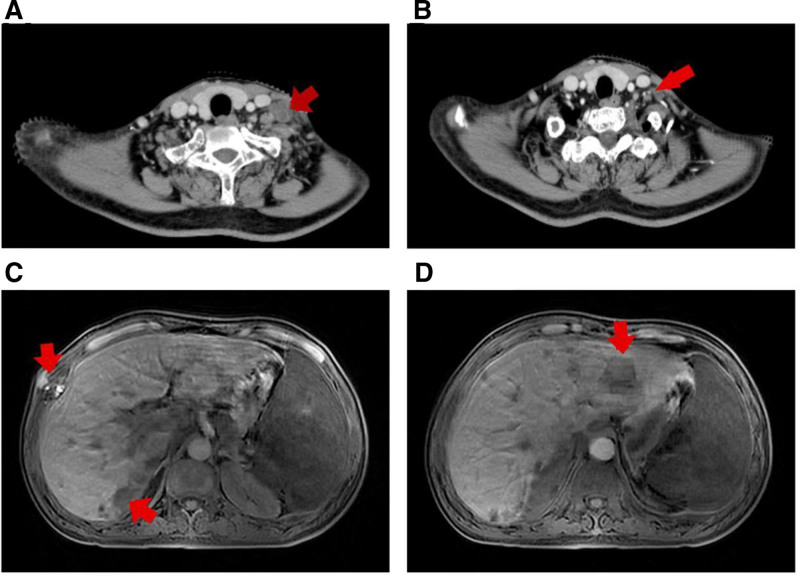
(A) Cervical lymph nodes before radiotherapy on July 27, 2022; (B) Cervical lymph nodes after stage I radiotherapy on August 18, 2022; (C, D) The transverse position T1 images (MRI) of liver and ribs metastases. MRI = magnetic resonance imaging.
